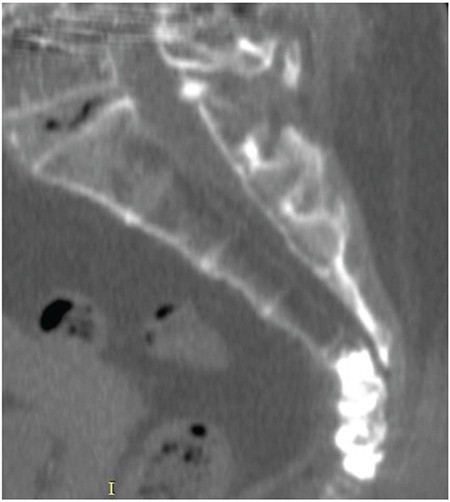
Computed tomography images after coccygeoplasty.
